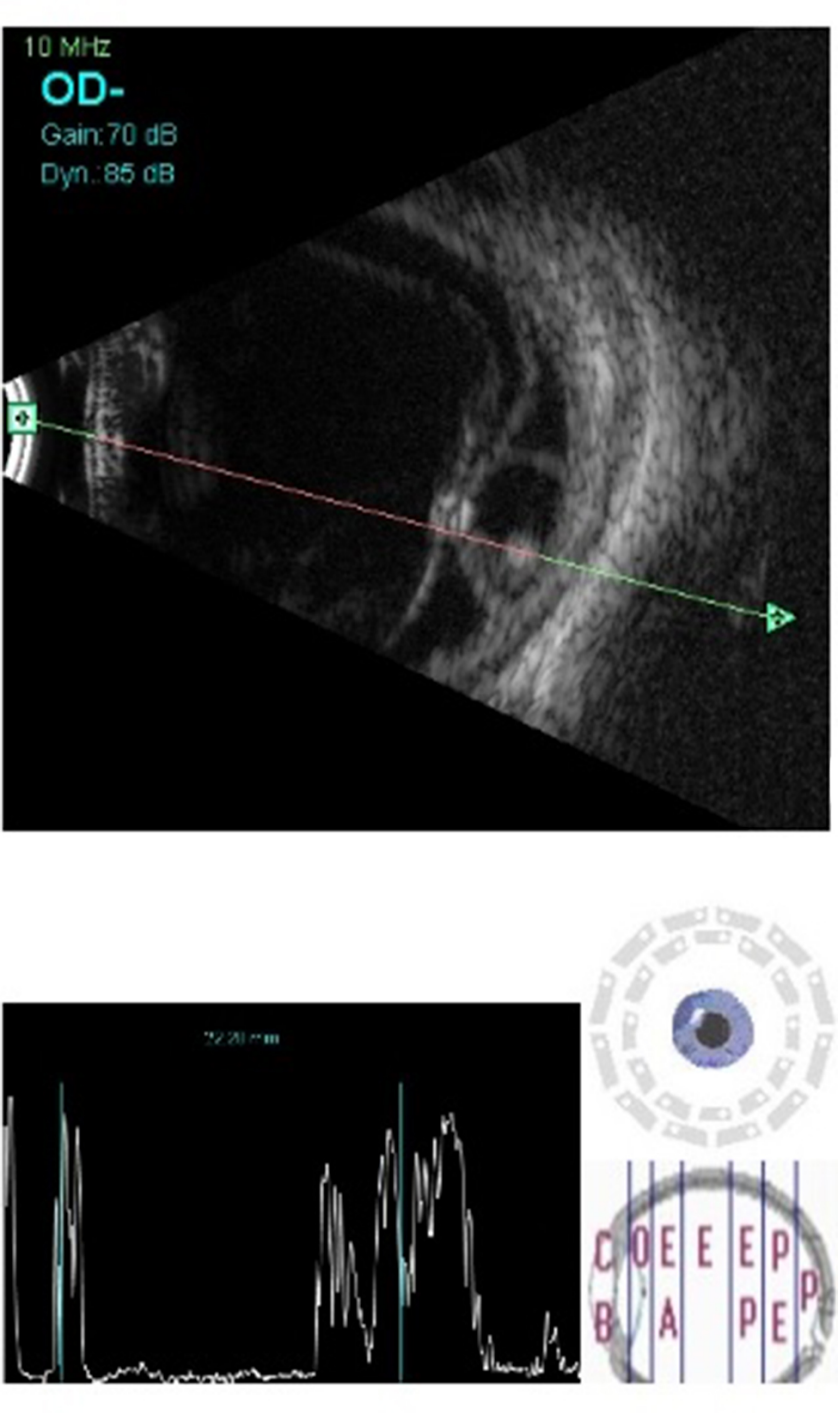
B-scan ultrasound of the posterior segment of the right eye showing inferotemporal retinal detachment with a well-defined subretinal cyst having high amplitude, dot-like echo suggestive of scolex. Hyperechoic dot with high amplitude spike in vitreous cavity suggestive of another scolex
